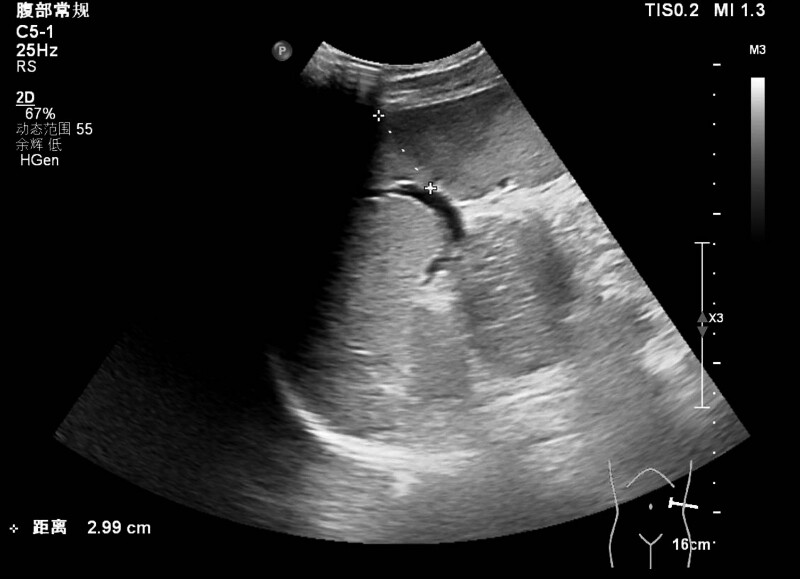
Ultrasound showing a hypoechoic wedge-shaped region of splenic tissue.
